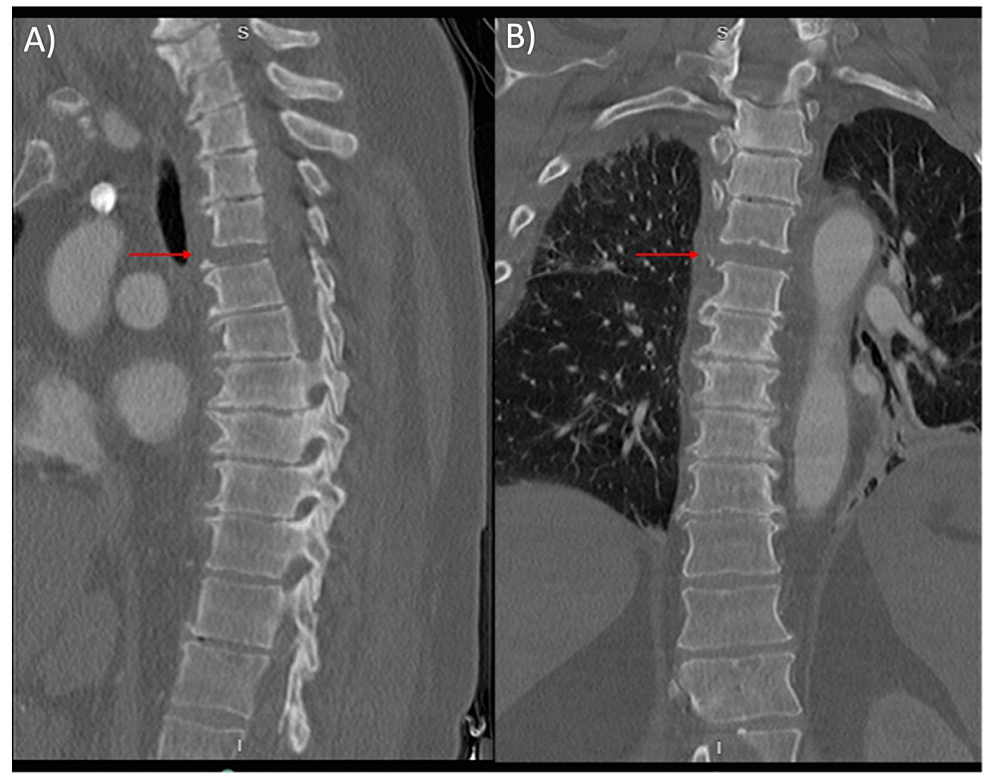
Sagittal (A) and Coronal (B) CT of Thoracic Spine T4/5 fracture-disruption with retrolisthesis of T4 on T5 as indicated with red arrows.
